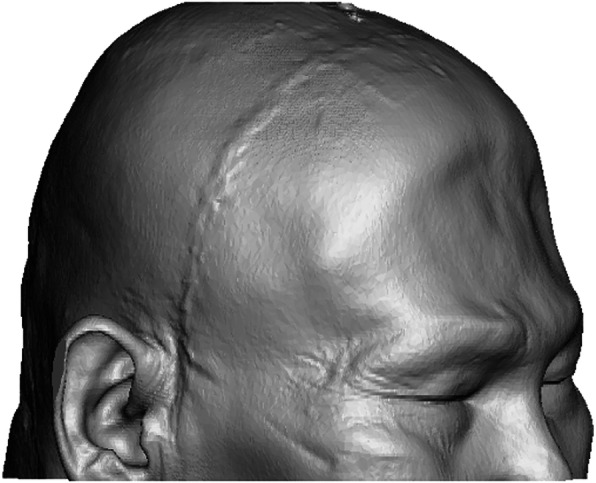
An accurate and detailed 3D skin surface rendering of the patient’s head, based on CT data
